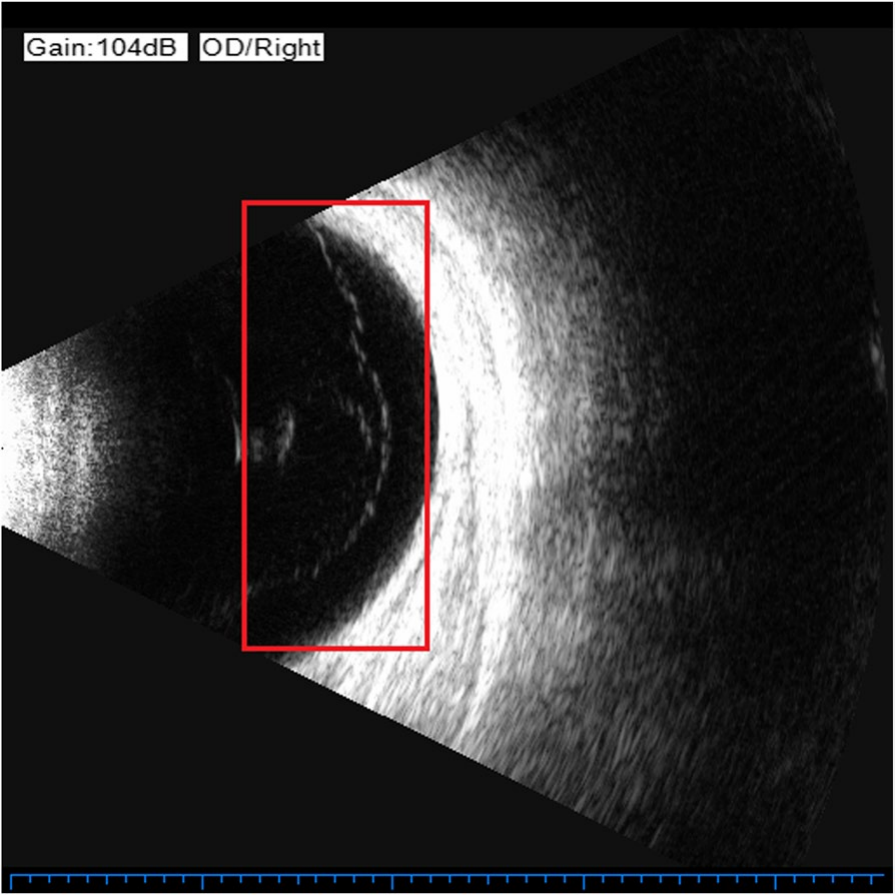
PVD fundus ultrasound image
